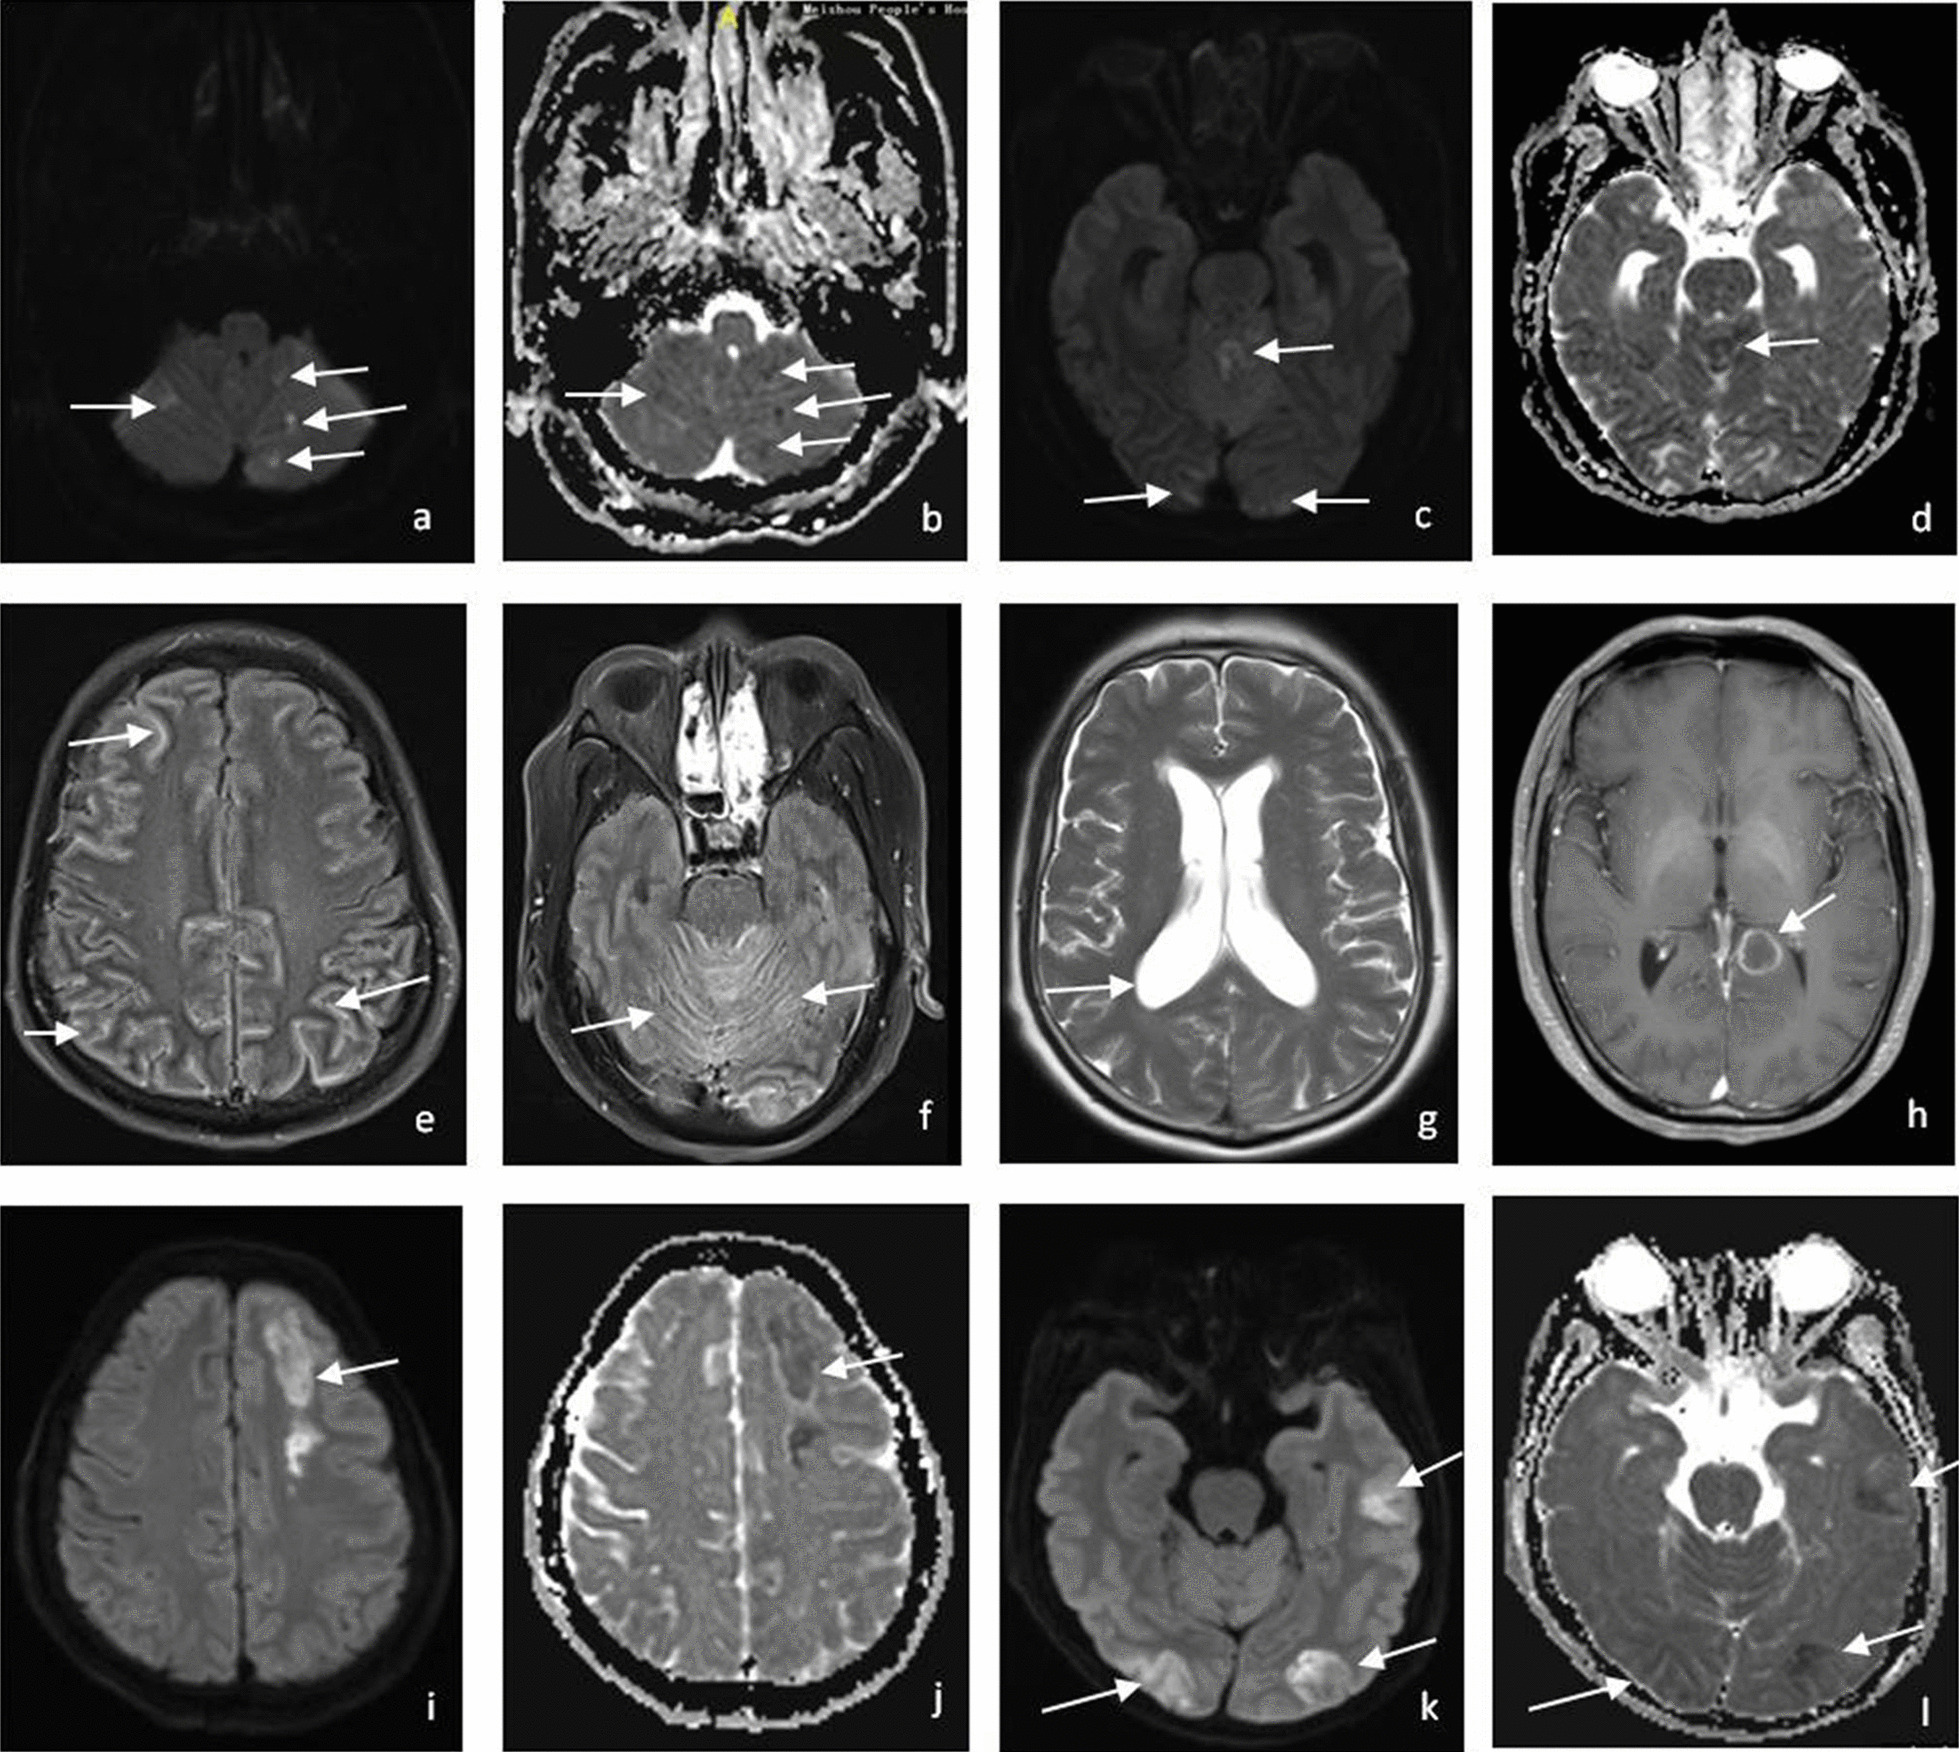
Representative MRI findings in HIV-negative patients with CM. A 64-year-old man with HIV-negative CM. a–d DWI and ADC map showing multiple lacunar infarction in cerebellar vermis, bilateral cerebellar hemispheres and bilateral occipital lobe. e, f Gadolinium (Gd)-enhanced FLAIR showing meningeal enhancement of cerebral cortex and brain stem and cerebellum (posterior fossa exudates). g T2WI showing ventricular dilatation (hydrocephalus). h Another patient with HIV-negative CM. Gadolinium (Gd) contrast enhanced T1WI showing remarkable ring enhancement in the posterior inferior region of left splenium of corpus callosum (cryptococcoma). i–l The third patient with HIV-negative CM, DWI and ADC map showing multiple large infarctions at the left frontal, left temporal, bilateral occipital lobe
